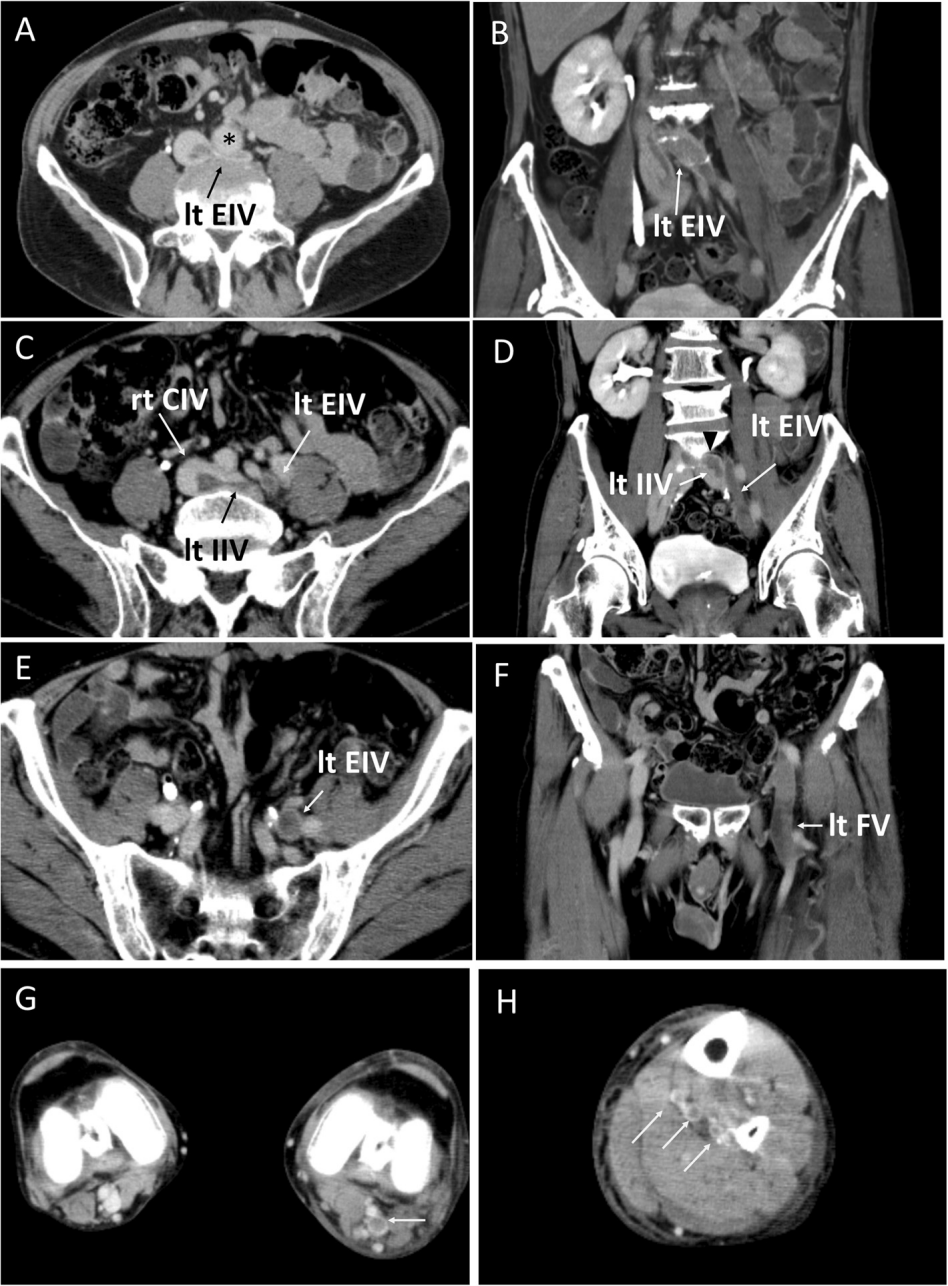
Multi-detector computed tomography (MDCT) on admission. A and B. Transverse plane (A) and coronal plane (B) at the ilio-cava junction level. Left external iliac vein (lt EIV) branches off directly from the inferior vena cava. The left EIA is compressed by the abdominal aorta (*) with thrombus; C to E. Transverse plane (C and E) and coronal plane (D and F) at the iliofemoral vein level. Lt EIV, right common iliac vein (rt CIV), left internal iliac vein (lt IIV) and left femoral vein (let FV) have thrombi; G. left popliteal vein thrombus (white arrow); H. thrombi of left lower leg veins/soleal veins (white arrows).
